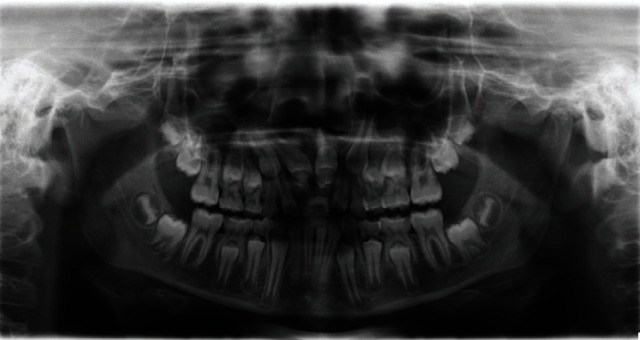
Orthopantomogram (OPG) at the age of eleven: the retention and the double formation of the central incisors can be seen.
